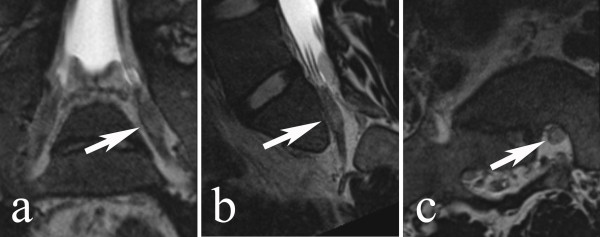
Magnetic resonance images (T2 weighed CCI SPACE sequence) of the left S1 spinal nerve at the level of the dorsal root ganglion (arrows). 1. Coronal view, 2. sagittal view and 3. axial view. All views are reconstructed projections parallel (coronal and sagittal) or perpendicular (axial) to the length axis of the nerve.
